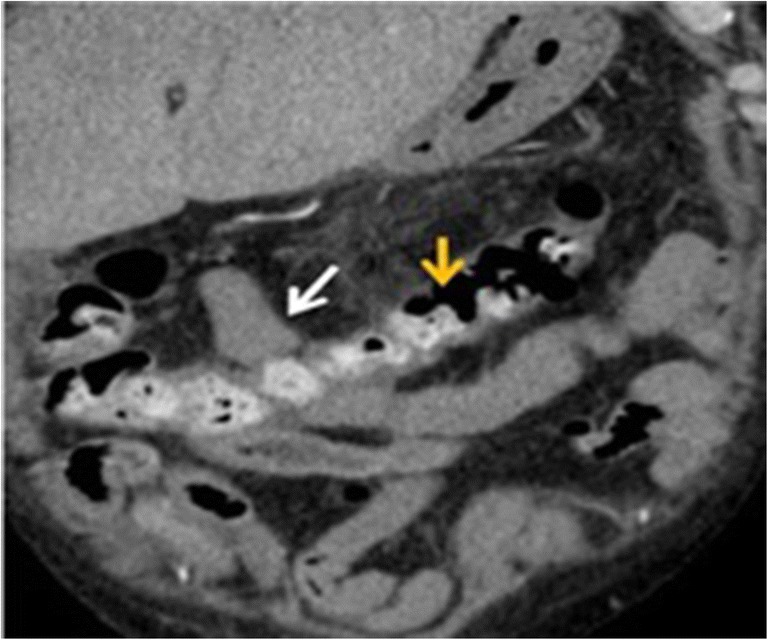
Bowel wall enhancement. Coronal contrast-enhanced CT shows “pale arterial ischaemia” with absent mural enhancement in a segment of small bowel (white arrow). An adjacent segment of small bowel shows mucosal hyperenhancement of thick-walled small bowel (orange arrow), indicating bowel reperfusion injury
